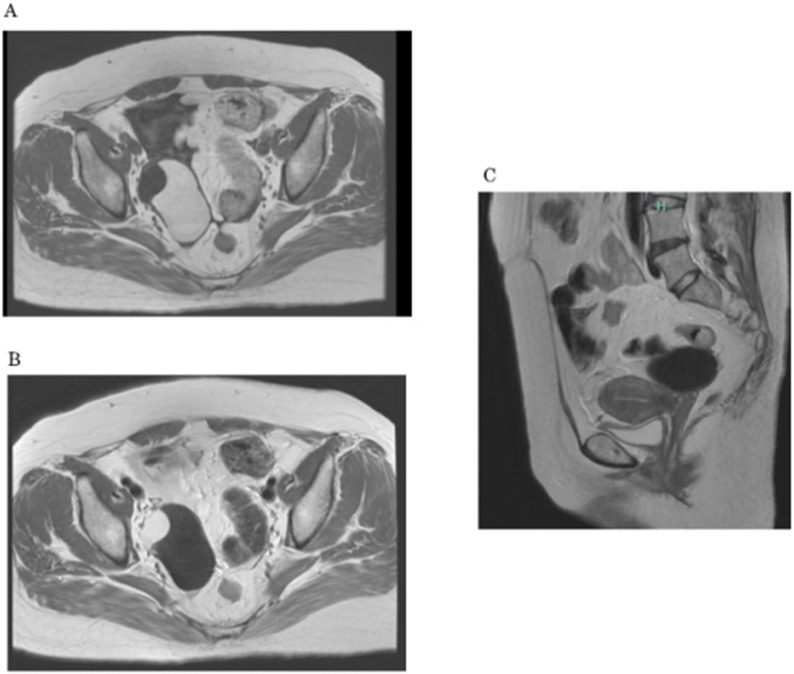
MRI images. A. T1-weighted imaging showed a 7-cm multilocular ovarian cyst, which exhibited regions of high and low signal intensity. B. A T2-weighted image is shown. C. T1-weighted sagittal imaging showed that the ovarian cyst was located behind the uterus.
